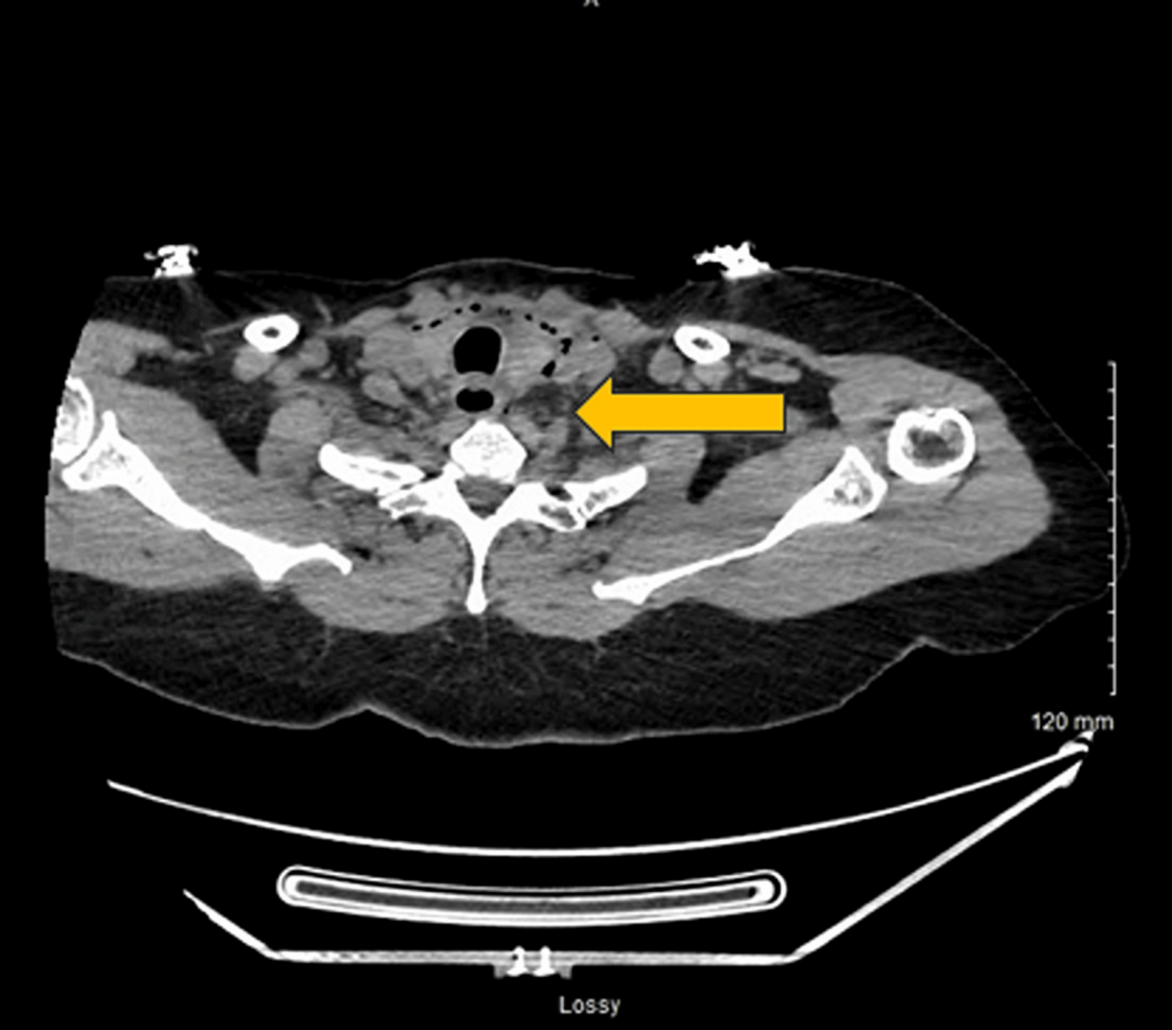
Repeat Computed tomography (CT) Neck Improvement in the air in the soft tissues of the neck.
